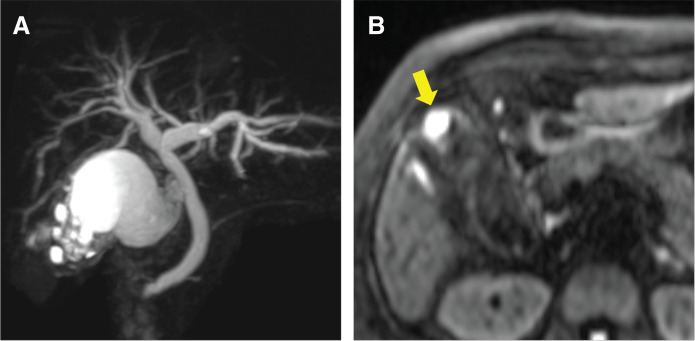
Magnetic resonance imaging findings. (A) A Rokitansky–Aschoff sinus is observed, and gallbladder adenomyomatosis is suspected. (B) Diffusion-weighted imaging shows nodular thickening of the fundus of the gallbladder with restricted diffusion (yellow arrow).
